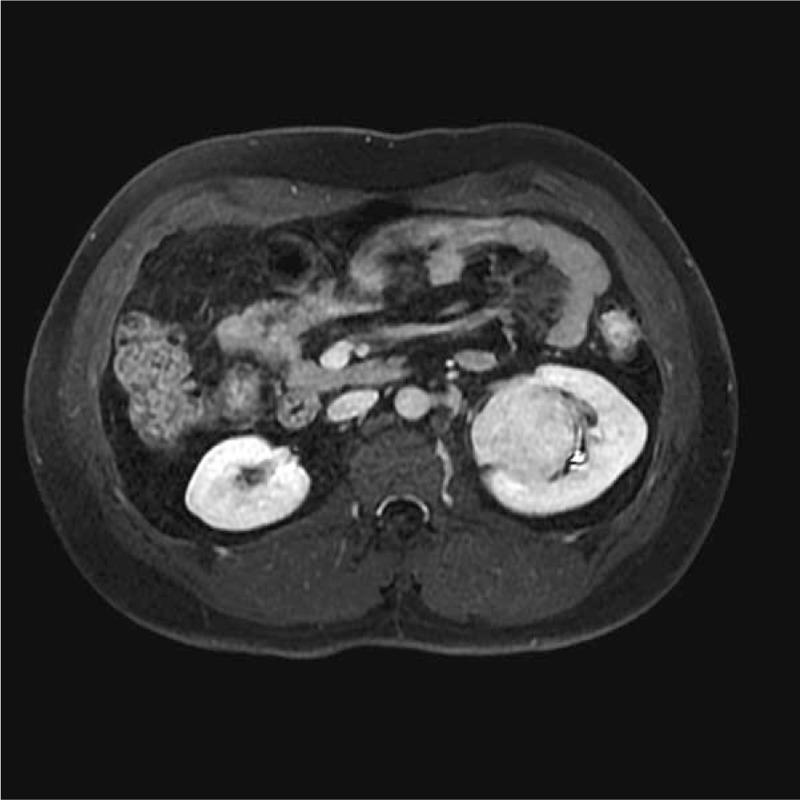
On dynamic contrast-enhanced magnetic resonance image, the delay phase image showed consistent enhancement.
